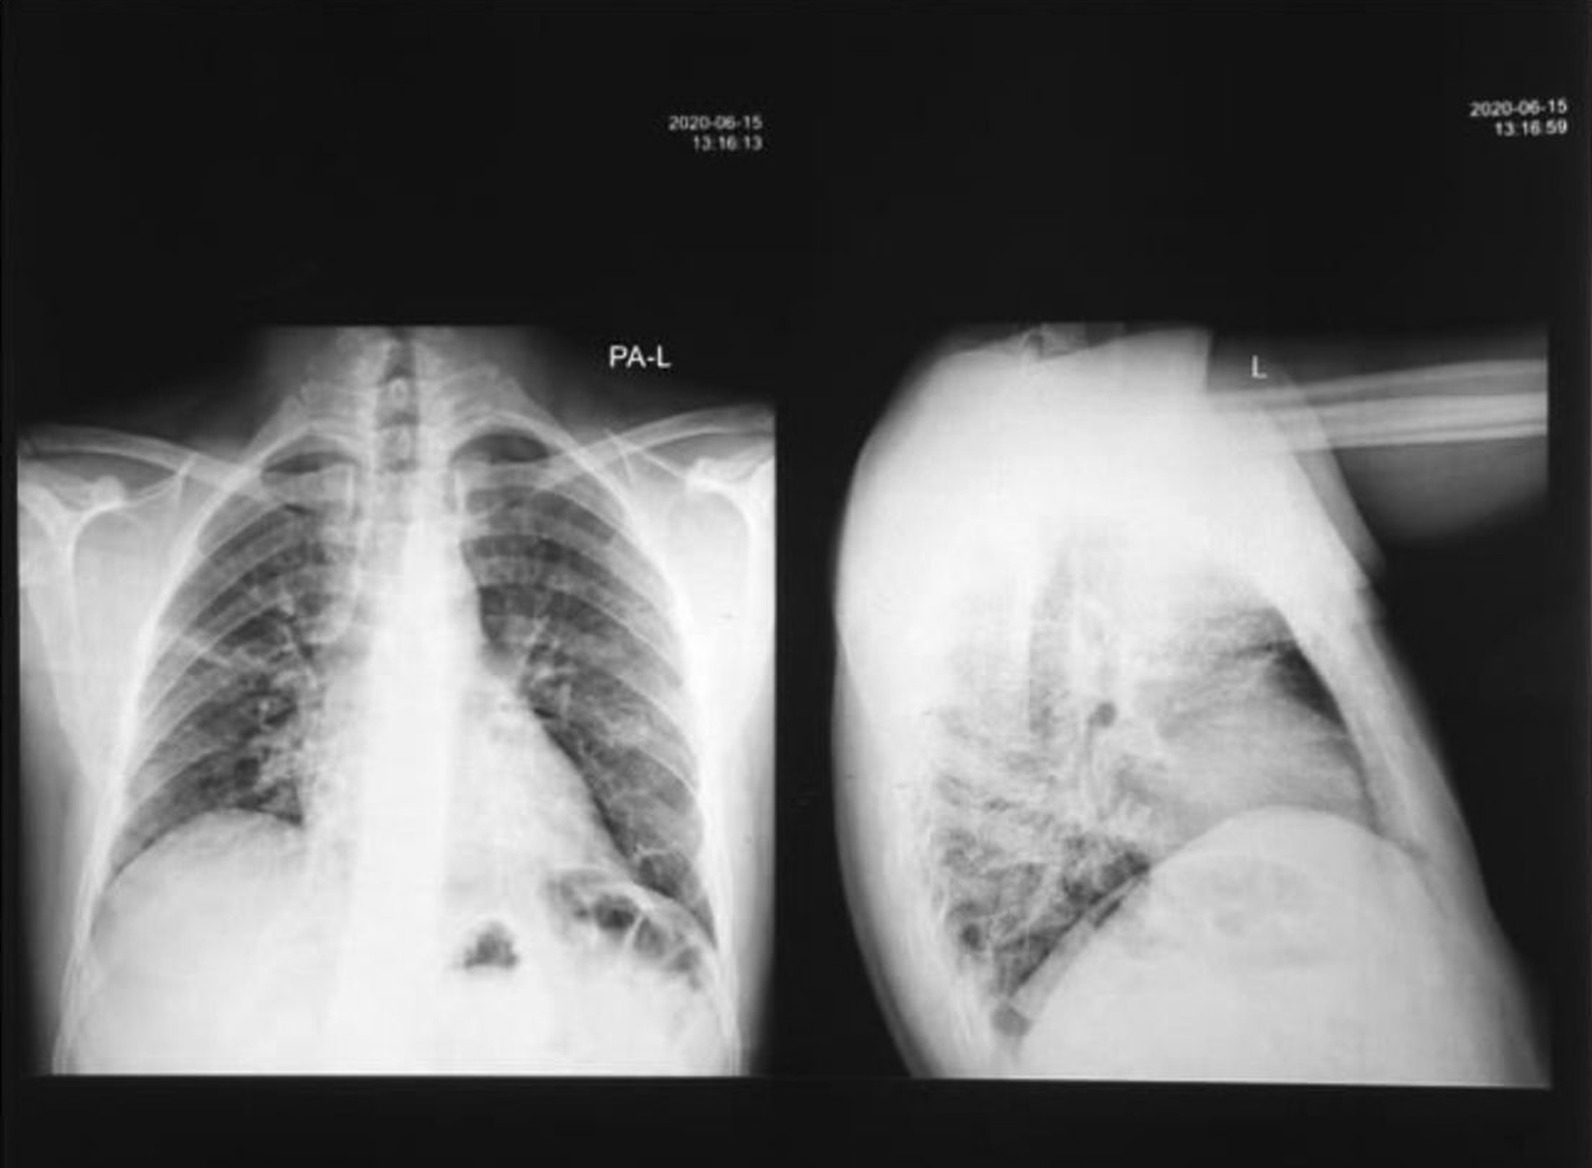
Anteroposterior/longitudinal chest X-ray (CXR AP/L). Imaging performed 10 days after COVID-19 presentation shows patchy infiltrates with ground-glass opacifications
